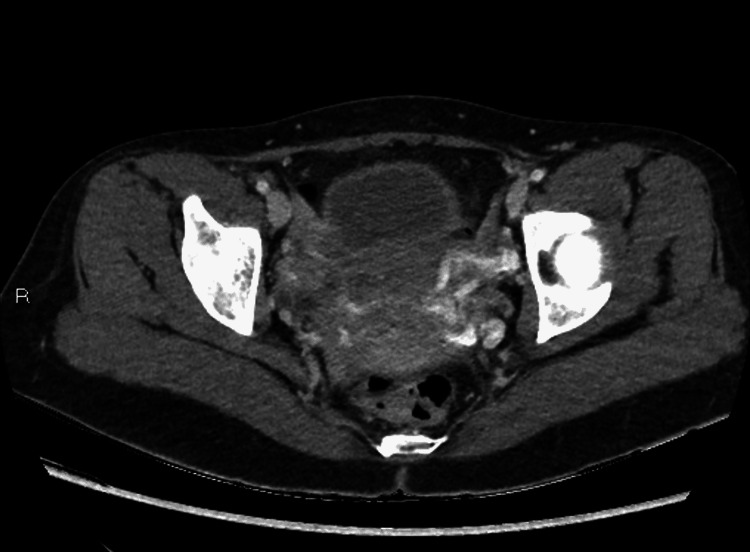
Axial images of the pelvic CT demonstrate asymmetrically dilated left adnexal/pelvic veins
